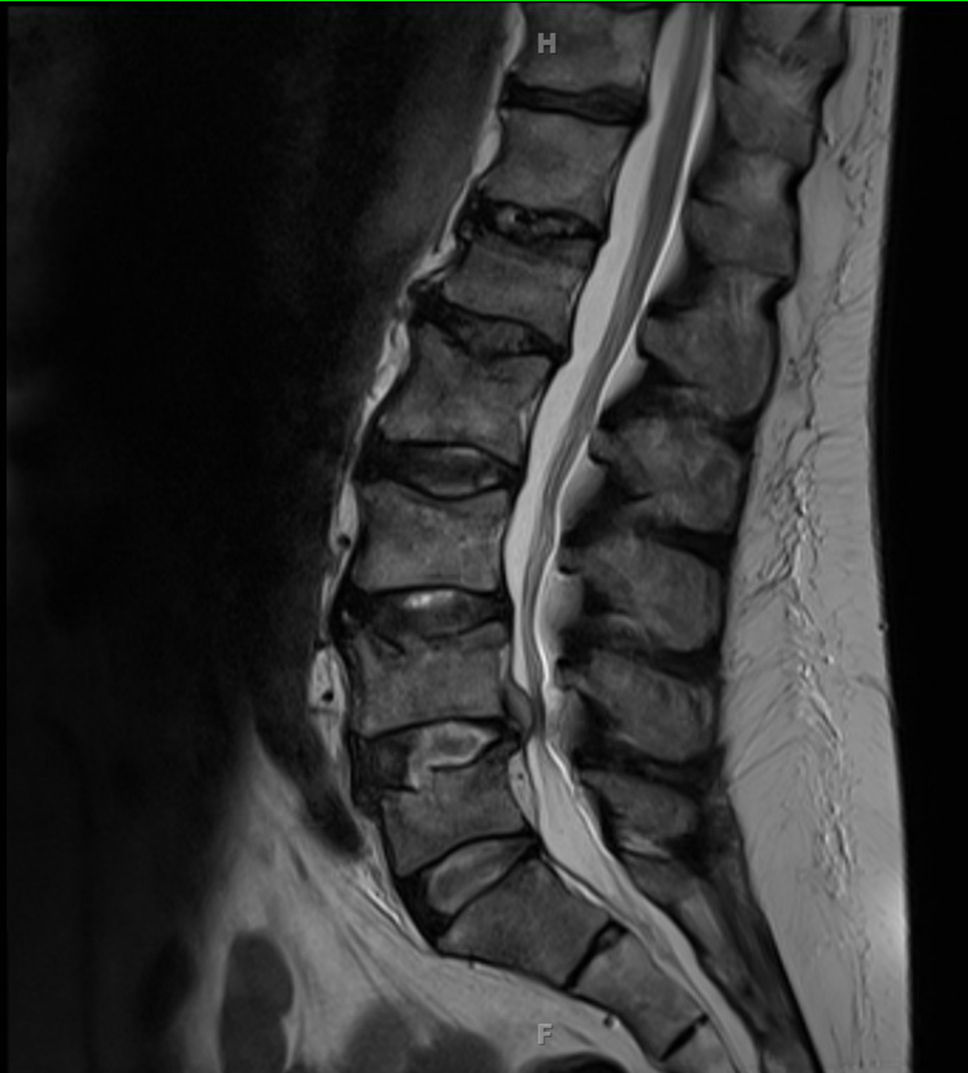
Lumbar MRI. MRI scan showing minimal to mild superior compression fractures from L1 through L5 with variable areas of enhancing oedema, with small lytic lesions throughout the lumbar spine and a large lytic lesion at the L5 vertebral body.
